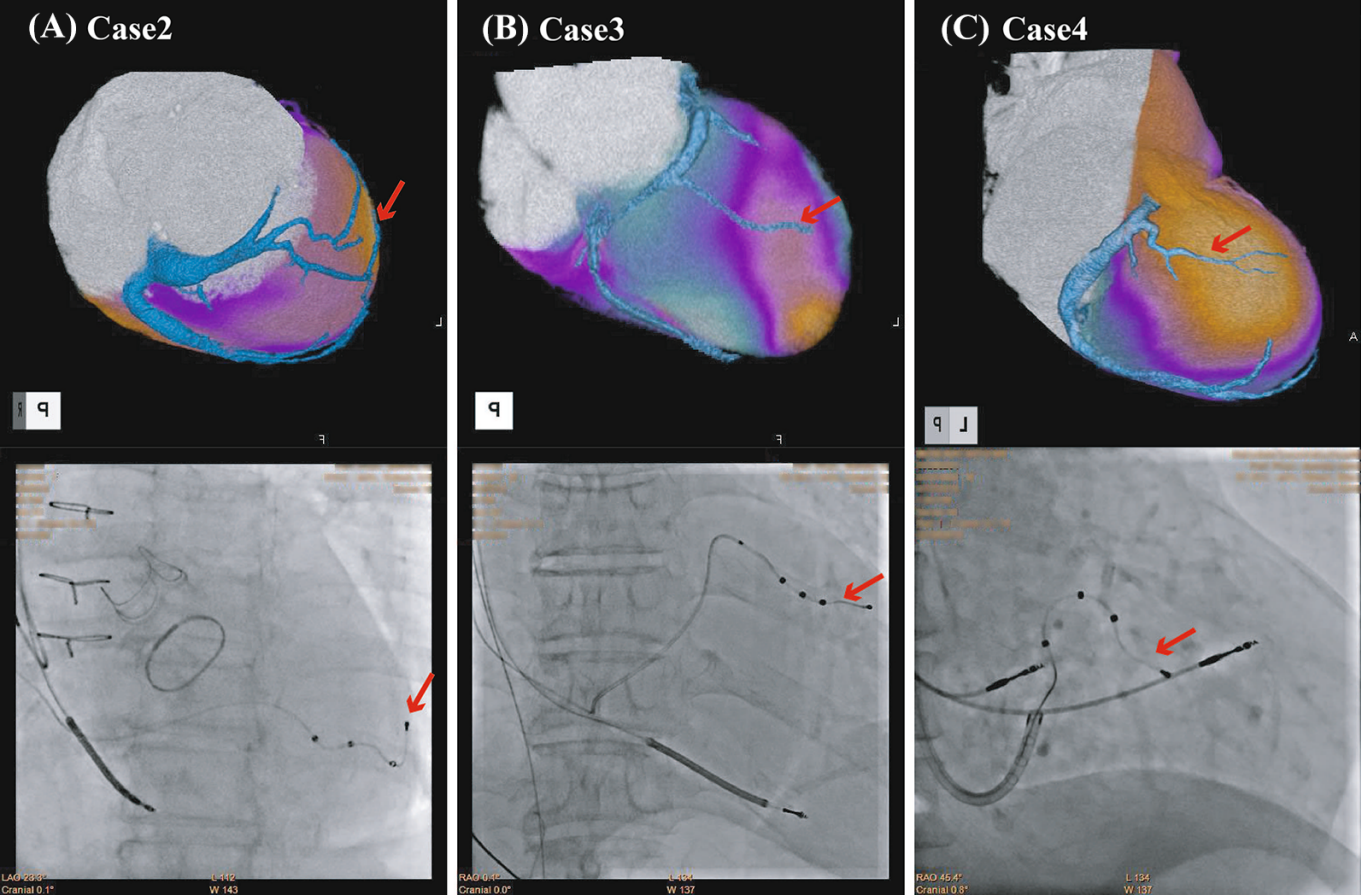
The fusion images of the myocardial perfusion image and coronary CT venogram (*upper panels*) in 3 cases. In all cases, the CRT pacing leads are appropriately located at viable myocardium (*lower panels)*. (A) Case 2, a 70-year-old female with idiopathic dilated cardiomyopathy (DCM) and a post-aortic valve replacement; (B) Case 3, a 60-year-old male with cardiac sarcoidosis; (C) Case 4, a 90-year-old male with DCM
